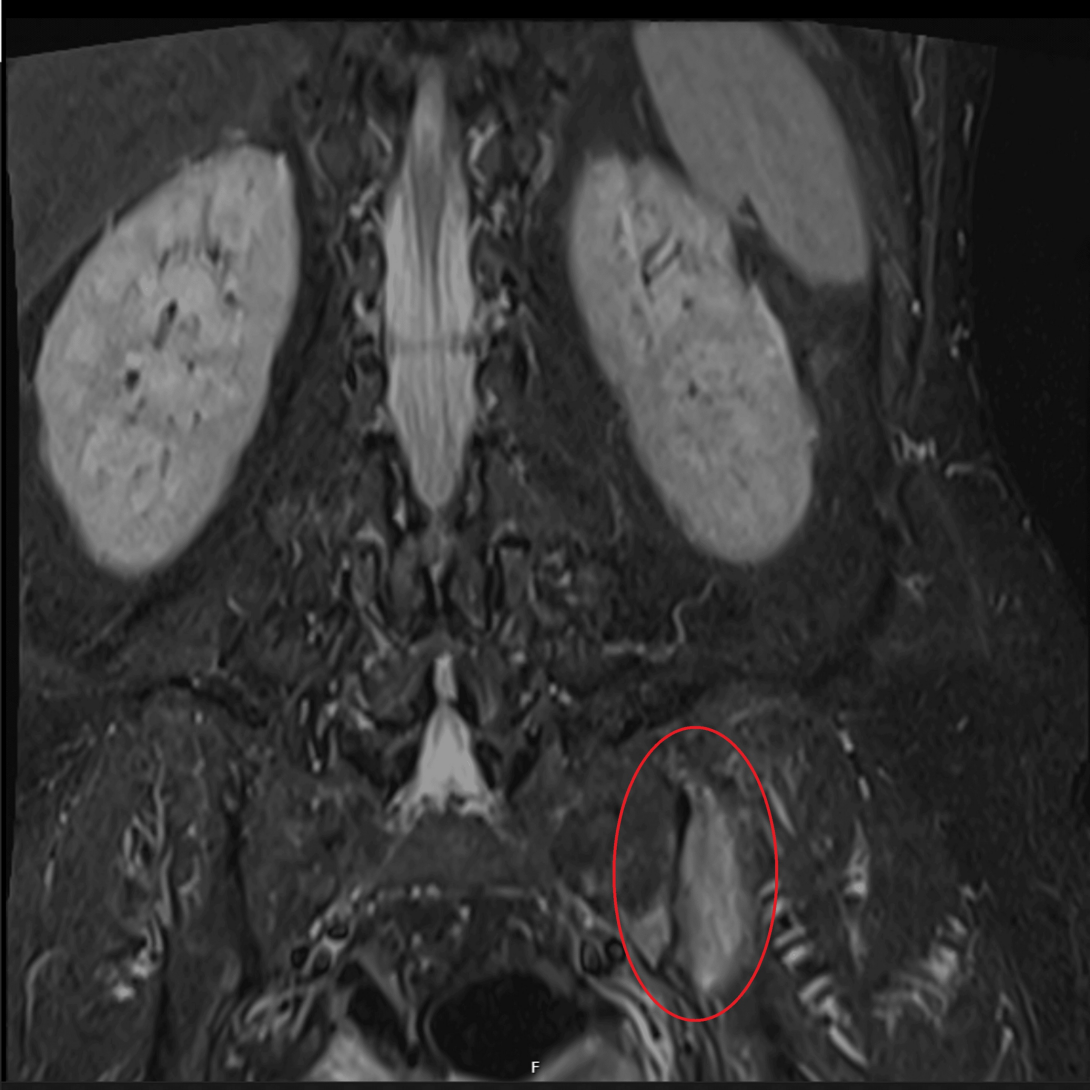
Lumbar MRI: asymmetric sacroiliitis (red circle).
